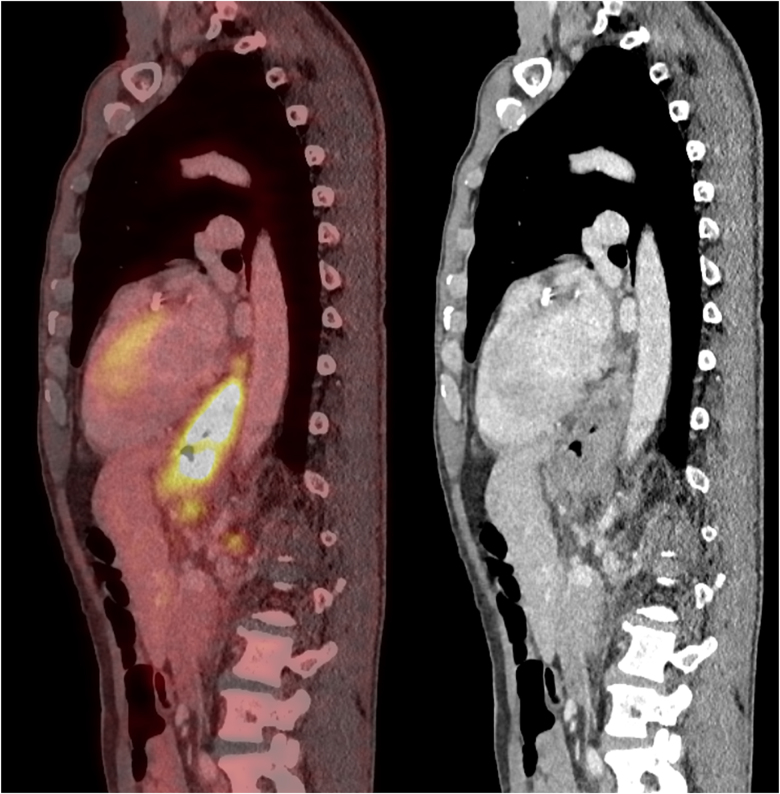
Siewert Type 2 primary lesion on sagittal PET/CeCT (CeCT = contrast-enhanced CT; CT = computed tomography; PET = positron emission tomography)
